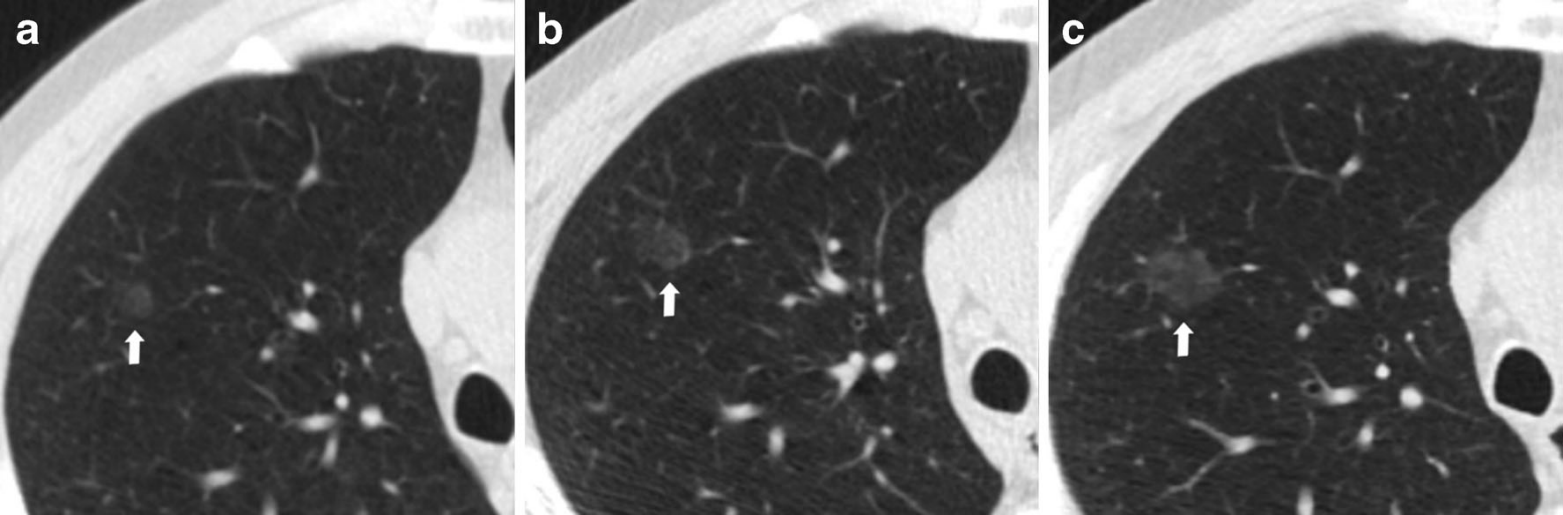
Slow-growing lung adenocarcinoma in a 61-year-old man. (a) Low-dose CT axial section in the right upper lobe shows a 6-mm ground-glass nodule (*arrow*). (b) Low-dose CT scan after 2 years shows an increase in size of the nodule to 10 mm (*arrow*). (c) After 4 years from the first low-dose CT scan, the nodule showed the same ground-glass attenuation but a progressive increase in size from 10 to 14 mm (*arrow*). Typically, early lung cancer presenting as non-solid nodules are slow-growing forms
